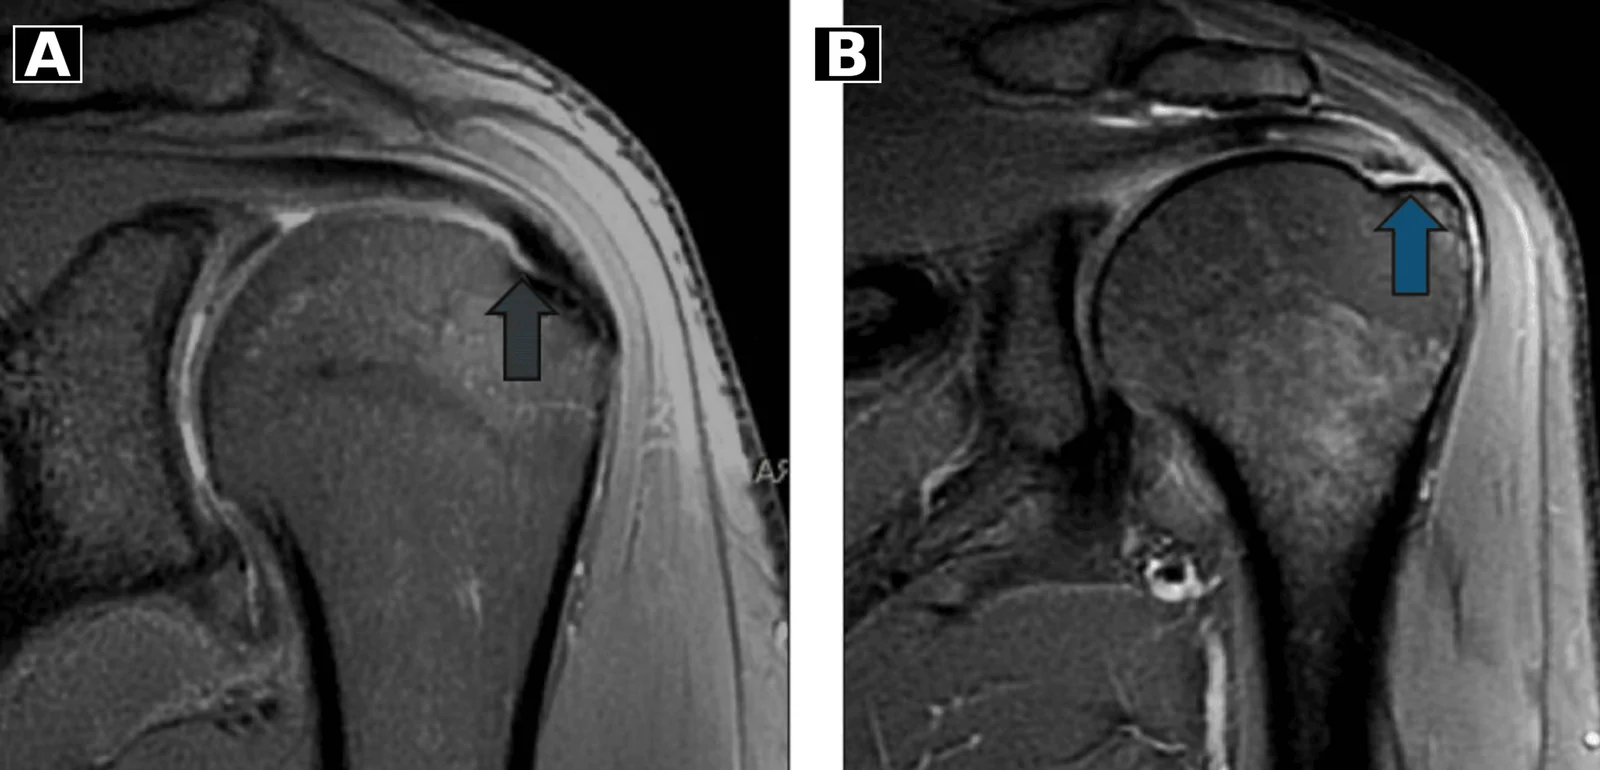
Ellman Grade I and Grade III partial-thickness supraspinatus tears on MRI A: coronal proton density fat-saturated MRI image demonstrates a low-grade partial-thickness tear of the supraspinatus tendon at the footprint, consistent with an Ellman Grade I tear (the arrow indicates focal superficial tendon fiber disruption involving less than 3 mm depth); B: coronal proton density fat-saturated MRI image demonstrates a high-grade partial-thickness supraspinatus tendon tear with deep fluid-signal extension at the footprint, consistent with an Ellman Grade III tear (the arrow indicates tendon fiber disruption involving more than 6 mm depth and more than 50% of tendon thickness).
